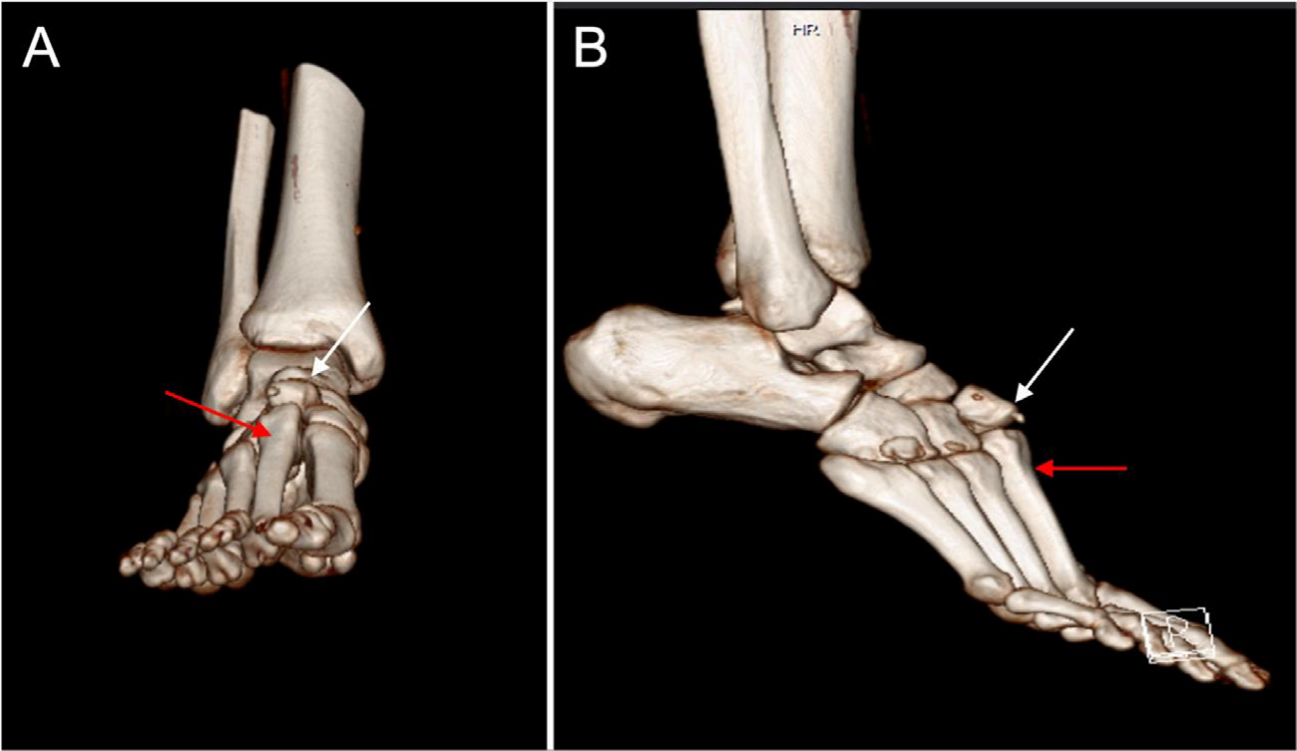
3D CT reconstruction images of the right foot prior to surgery. (A) Anterior view, and (B) Lateral view, showing a Lisfranc-type injury with dorsal dislocation of the intermediate cuneiform (white arrows) and second metatarsal (red arrows).
